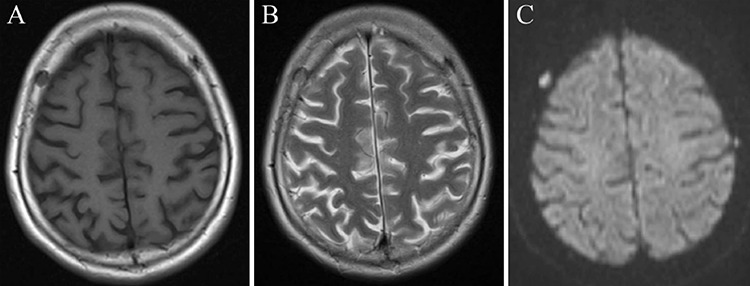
A-C. Cranial magnetic resonance imaging depicting multiple skull lesions with T1 hypointense signal (A), T2 slightly hyperintense signal (B), and diffusion-weighted imaging hyperintense signal (C).
